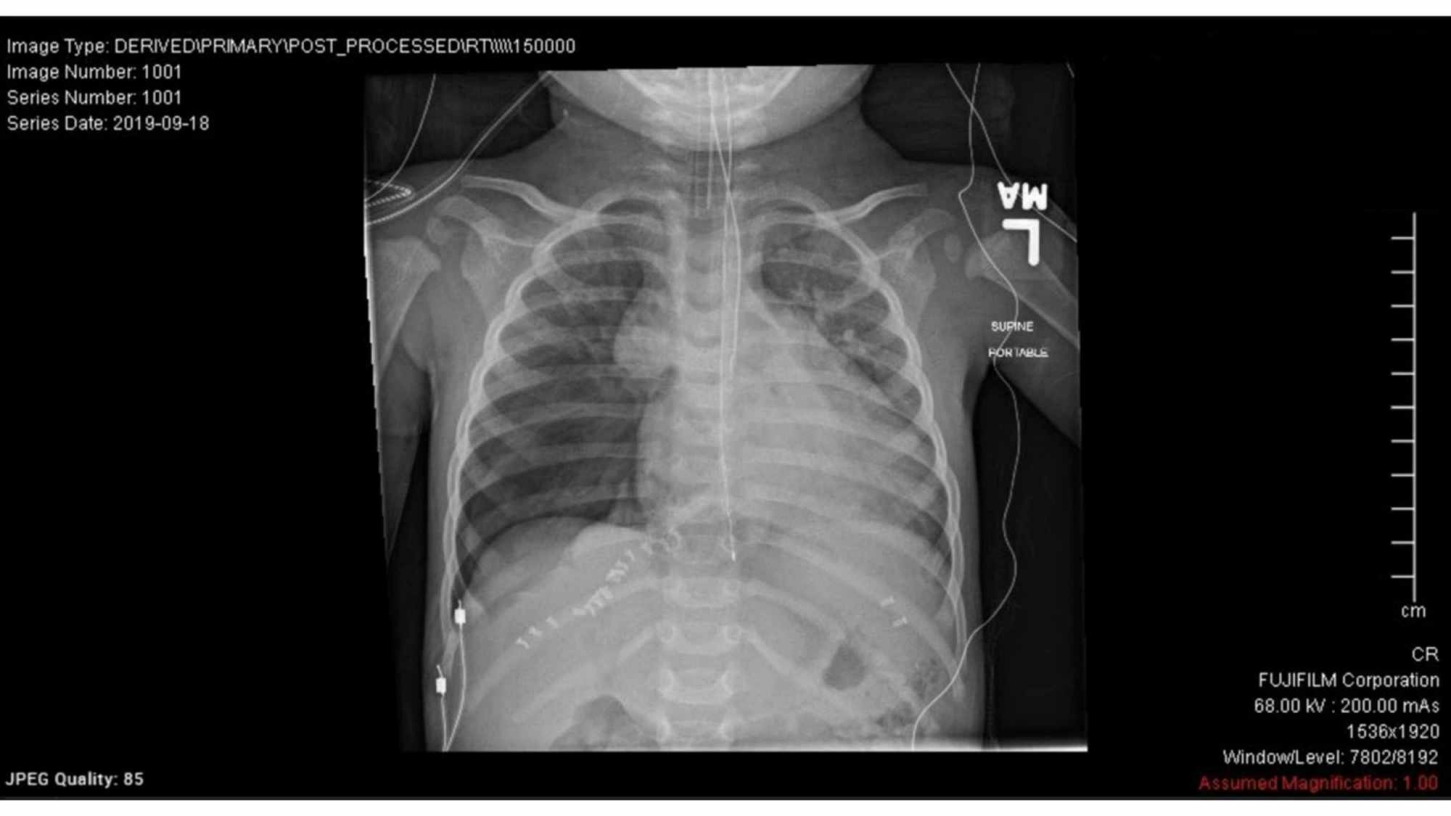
Intraoperative X-ray showing moderate right-sided pneumothorax status post hernia repair
